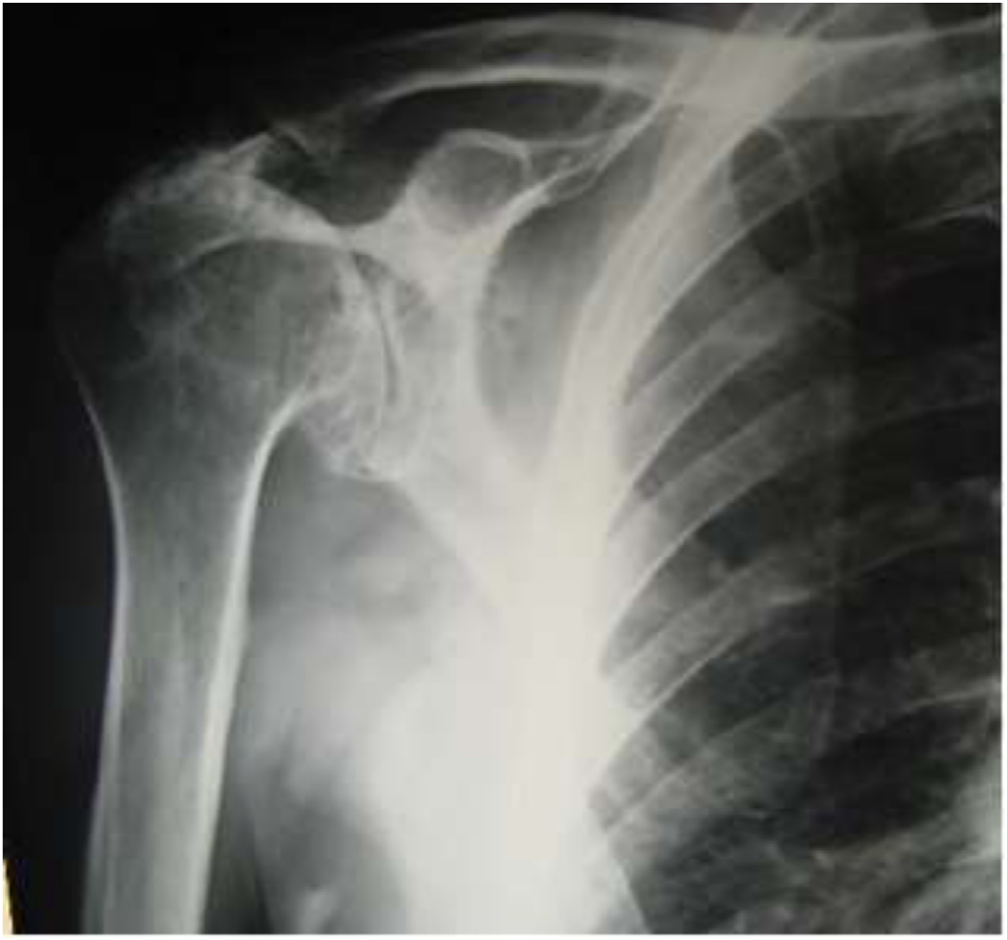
Preoperative x-ray showing the severe arthropathy.
